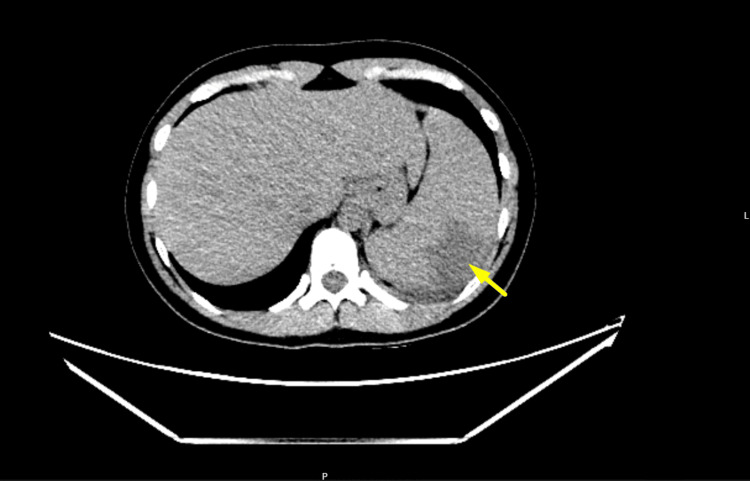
Cross-sectional contrast-enhanced CT of the abdomen Cross-sectional contrast-enhanced CT of the abdomen (portal venous phase) showing splenomegaly (13.3 cm) with a characteristic subcapsular peripheral wedge-shaped hypoenhancing area along the splenic dome (indicated by arrow), consistent with splenic infarction.
